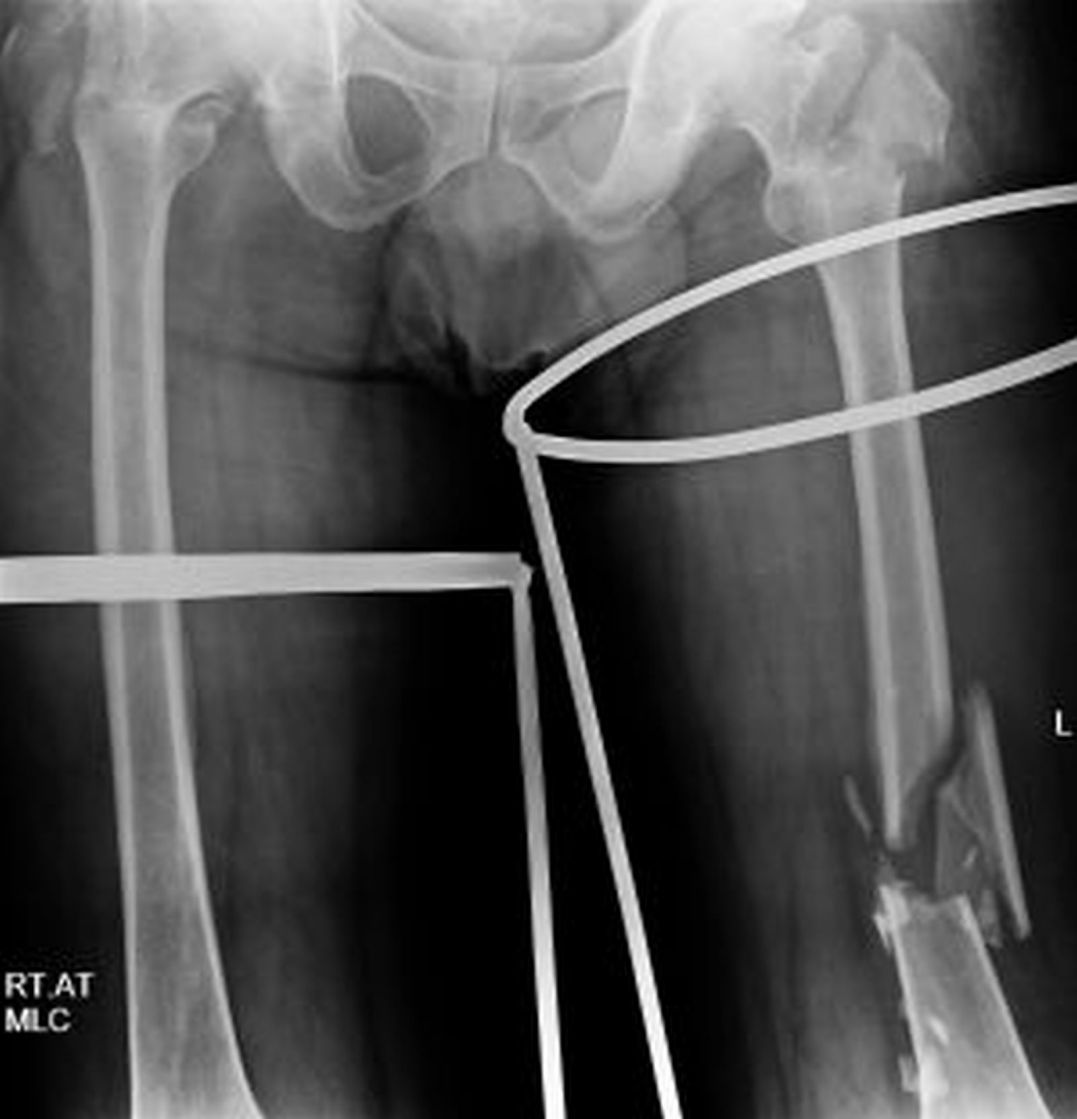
X-ray of the pelvis with both thighs showing comminuted left femoral shaft fracture
